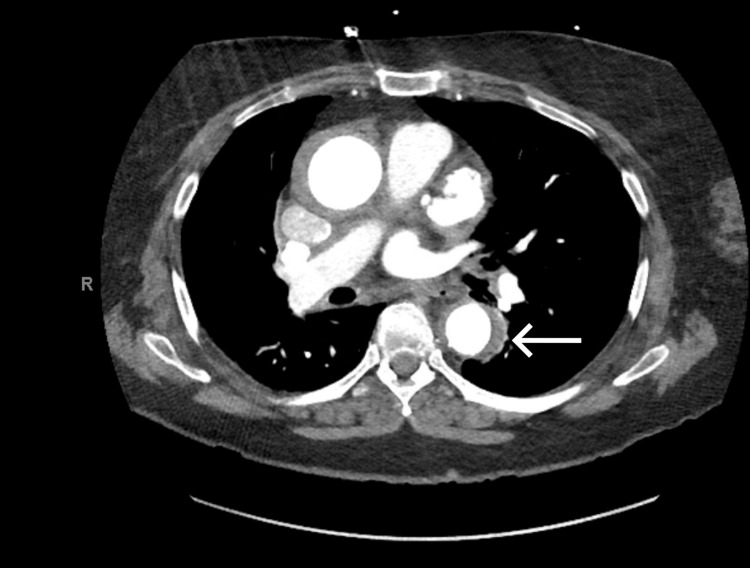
Axial computed tomography angiography (CTA) demonstrating absence of intimal flap. Axial CTA of the thorax demonstrates circumferential aortic wall thickening without evidence of an intimal flap or separation into true and false lumens. The absence of contrast within the thickened wall supports the diagnosis of intramural hematoma rather than classic aortic dissection.
